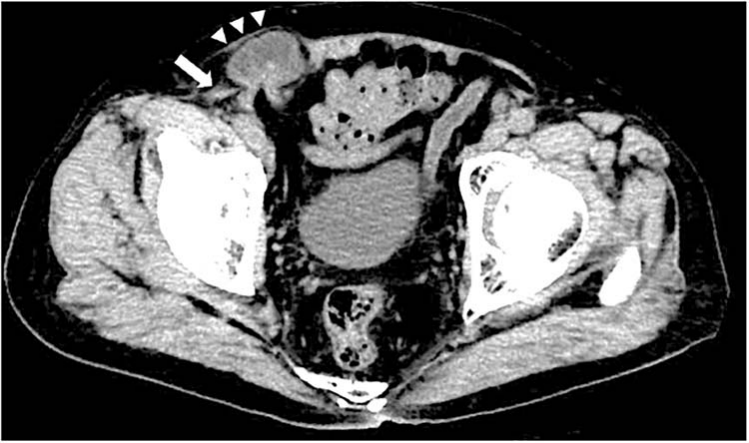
Contrast-enhanced CT scan; an incarcerated bowel was observed in the right inguinal region; the small bowel was incarcerated between internal and external oblique muscles; the white arrow indicates the internal oblique muscle, and the white triangles indicate the external oblique muscle.
